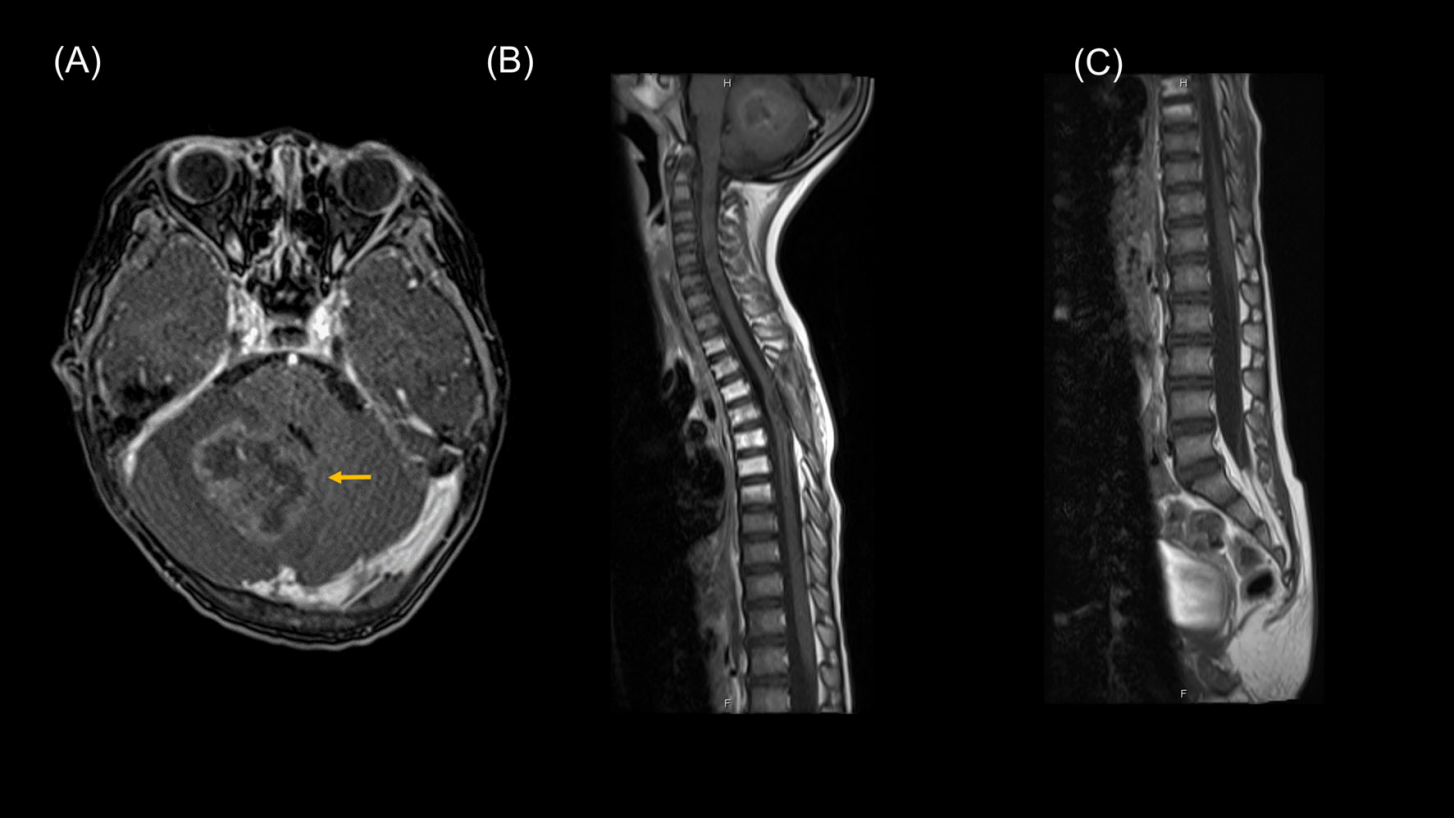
Gd‐T1w MRI at first relapse in the cerebellum. No new lesions were observed in the spinal cord. Subsequently, gross total resection was performed, and local radiotherapy was administered.
